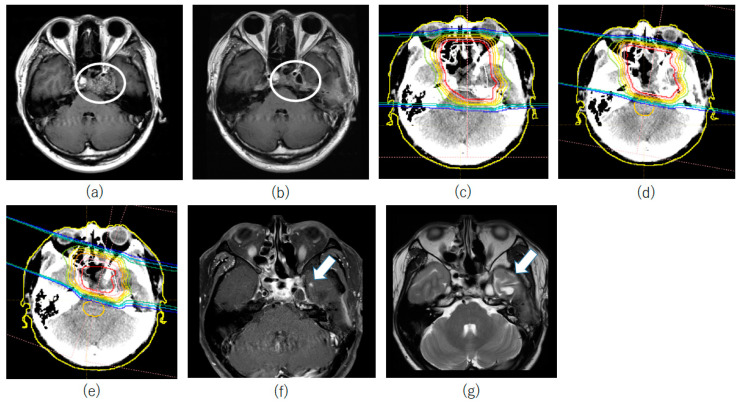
A case of temporal lobe radiation necrosis. (a) A tumor was found in contact with the left temporal lobe (white circle). (b) The tumor was grossly excised (white circle). (c) Irradiated field 0 to 39.2 Gy(RBE) in 28 fr (twice per day) including the tumor bed and surgical pathway. Dose distribution. The isodose lines represent 100–10% of the prescription dose from inside to outside. (d) Irradiated field 40.6 to 58.8 Gy(RBE) in 42 fr (twice per day) including the tumor bed and surgical pathway with a minimized radiation dose to the optic nerve, optic chiasm, temporal lobe, retina and brainstem. (e) Irradiated field 60.2 to 78.4 Gy(RBE) in 56 fr (twice per day) with a minimized radiation dose to the optic nerve, optic chiasm, temporal lobe, retina and brainstem. The maximum dose to the retina is 50 Gy(RBE), that to the optic nerve and chiasm is 54 Gy(RBE) and that to the brainstem and temporal lobe is 60 Gy(RBE). (f) At 44 months after proton beam therapy, contrast-enhanced MRI showed a small contrast-enhanced lesion in the left medial temporal lobe (white arrow). This spot was included in the irradiated area at boost. (g) There was mild edema around the contrast-enhanced lesion (white arrow).
